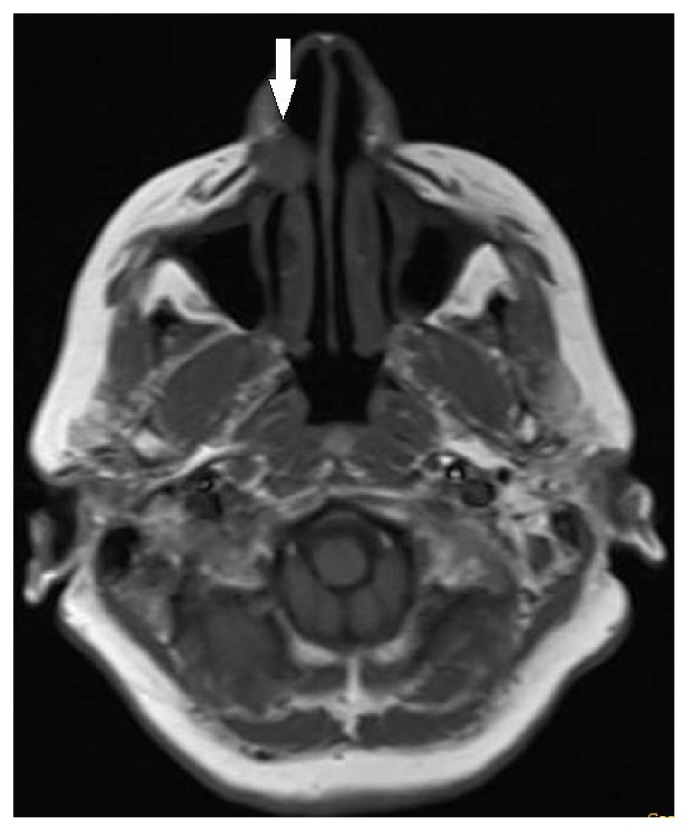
The axial T1-weighted MR image shows the lesion with a homogeneous hypointensity (arrow).
